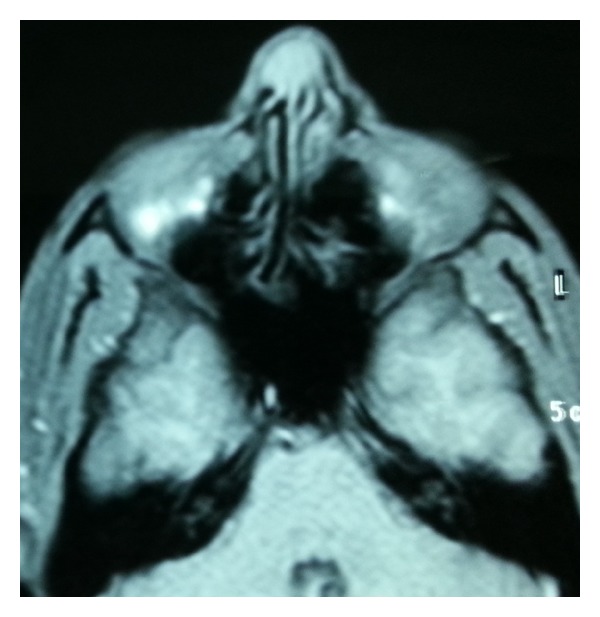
Cystic mass in T2-weighted cranial MRI on the right side of the nasal dorsum.
